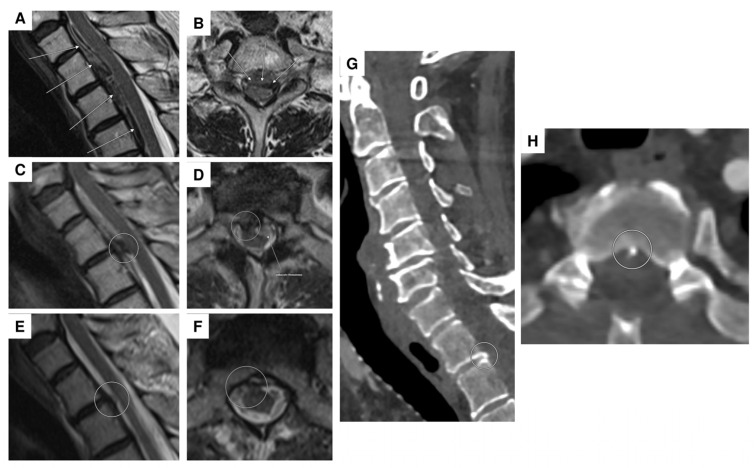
Spinal MRI of acute and subacute epi-/subdural hemorrhage with a suspected thrombosed perimedullary aneurysm at the T1/2 level. Initial MRI showed an acute epi-/subdural hemorrhage extending ventrally from levels C1 to T4, with maximal prominence at level T1 (arrows; T_2_-weighted sequence in the sagittal (A) and transversal planes (B)). The follow-up MRI five days later ((C): sagittal T_2_-weighted sequence; (D): transversal contrast-enhanced T_1_-weighted sequence) revealed ongoing (subacute) epi-/subdural bleeding from levels C7 to T3 ventrally (the arrow in (D)) and a newly suspected thrombosed aneurysm with slight wall enhancement at the T1/2 level, located perimedullarily and ventrolaterally on the right (circles in (C,D)). Follow-up MRI two weeks after the initial scan ((E,F): sagittal and transversal T_2_ -weighted sequence) revealed regression of the hematoma, with no remaining evidence of the aneurysm, suggesting probably complete occlusion (circled area). Spinal CT demonstrated a bony spur (circled area) at the dorsal margin of the intervertebral T1/2 level ((G,H): sagittal and transversal planes). Note: two DSAs were performed, with no vascular pathology detected.
